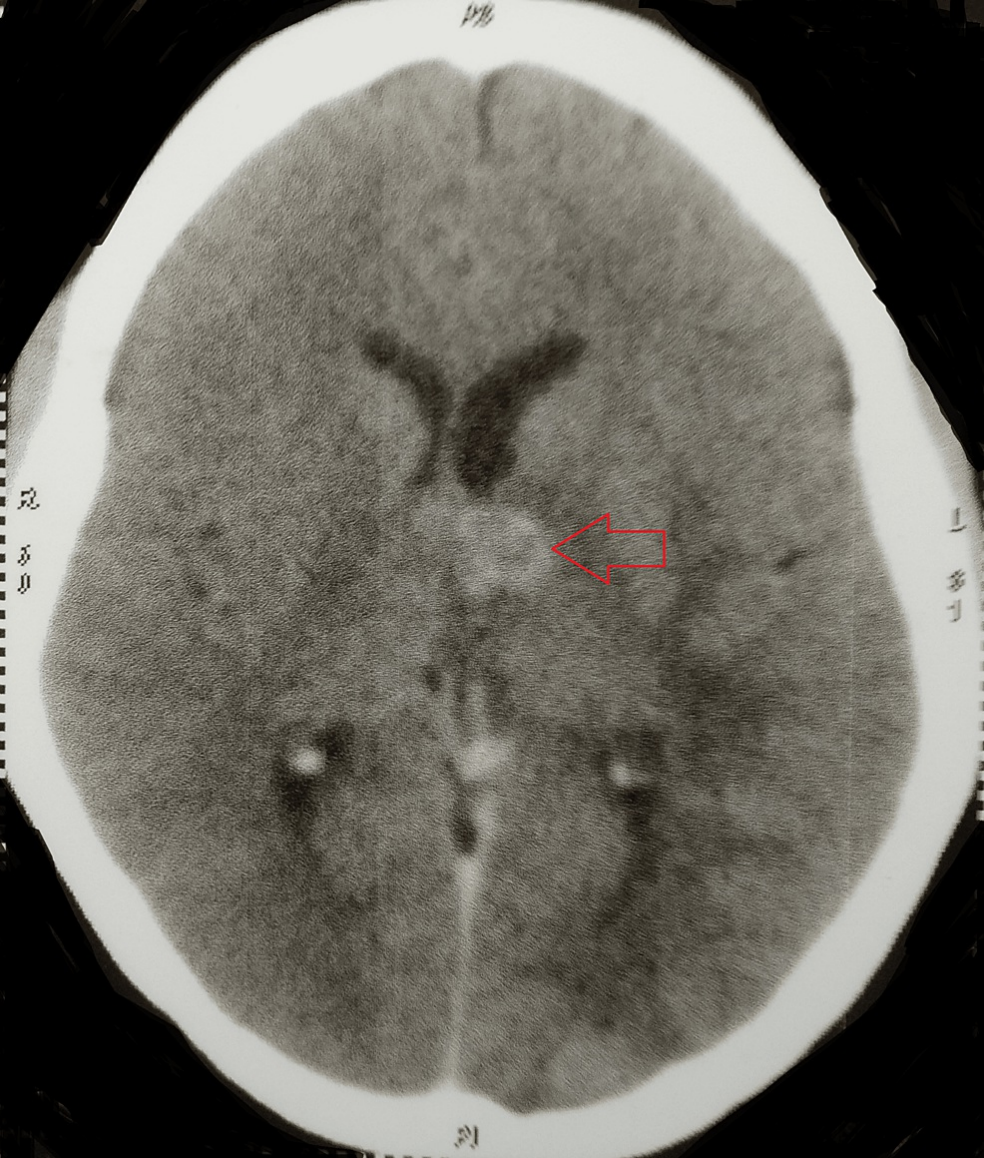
CECT of the brain showing a well-defined, discrete ring-enhancing lesion in the left thalamus CECT: contrast-enhanced computed tomography
